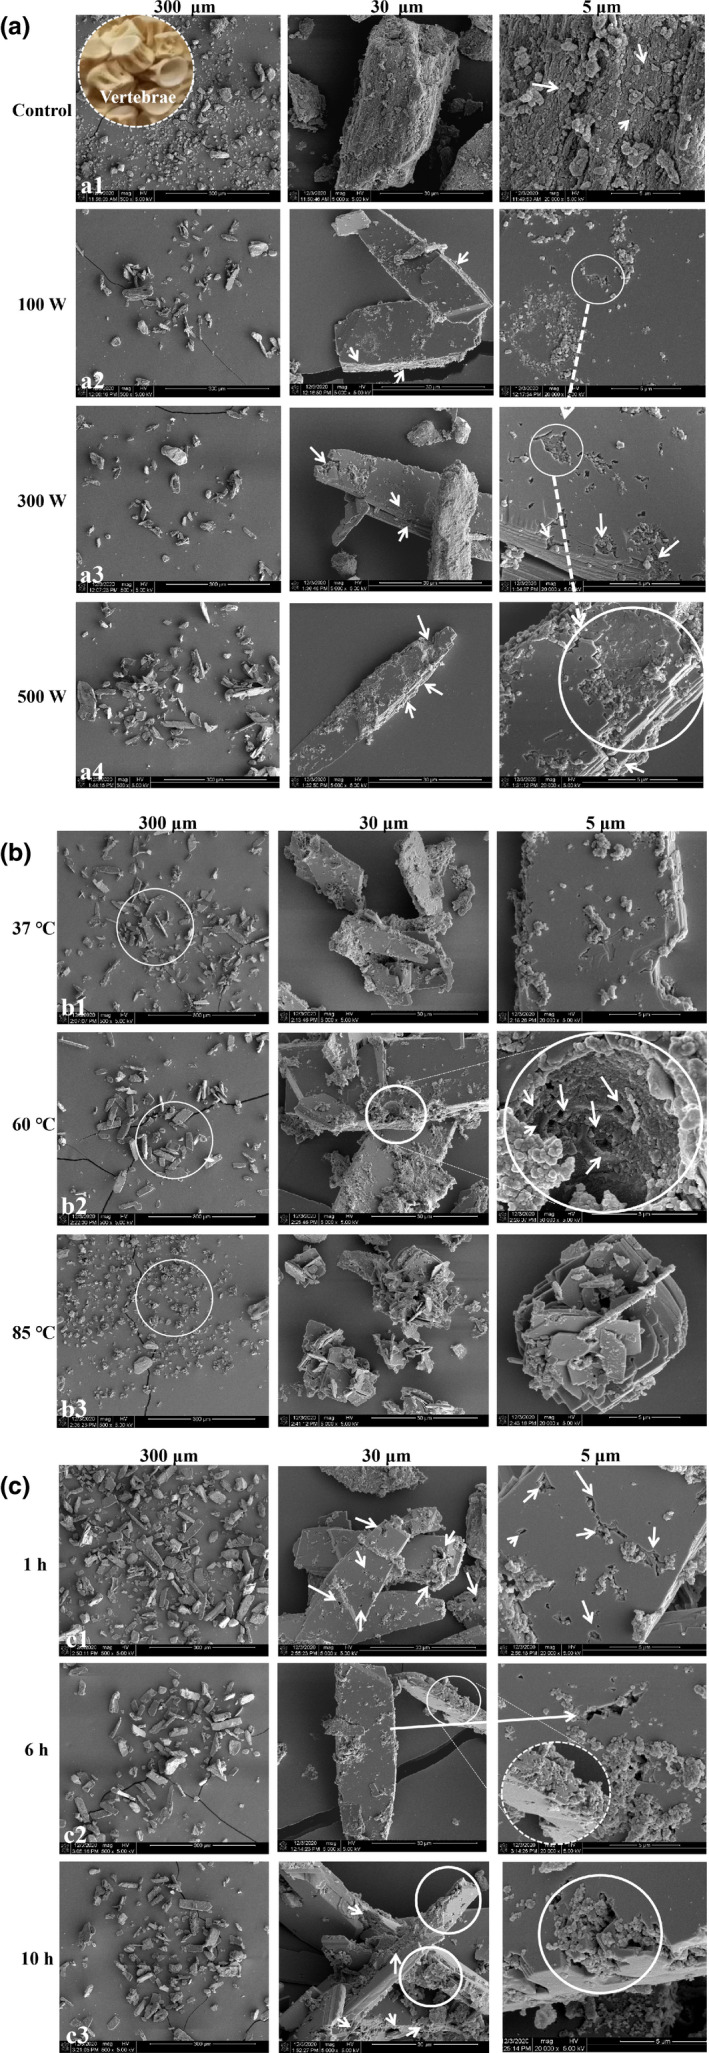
Effects of ultrasonic treatment on the surface morphology of MFPs. a, b, and c represent ultrasonic power, temperature, and time, respectively. Arrows show the structural changes in density and aperture
